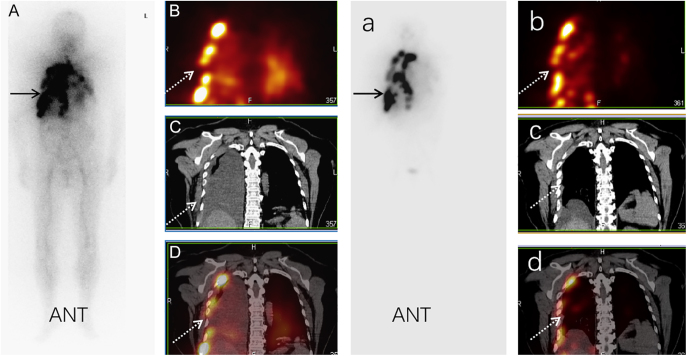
Representative case with a PR. The patient was a 51-year-old woman (case 3) who underwent near-total thyroidectomy with radical right neck dissection for PTC followed by ^131^I therapy at a dose of 7.4 GBq (200 mCi) for pulmonary metastases. A ^131^I-WBS performed 5 days after oral administration of ^131^I revealed diffuse uptake in the chest (A, solid black arrow). A ^131^I-SPECT/CT was then performed to localize the lesions in the chest and indicated uptake of ^131^I in multiple pleural and lung nodules, combined with severe MPE (B, C, D). The patient was retreated with 7.4 GBq of ^131^I for multiple metastases 4 months after the first ^131^I treatment. Post-treatment ^131^I-WBS and ^131^I-SPECT/CT fusion images showed markedly less ^131^I uptake in multiple pleural nodules, indicating a reduction in the lung nodules and MPE (B, C, D). At the same time, the stimulated Tg level decreased from 11,553.00 ng/mL to 1142.00 ng/mL. The patient achieved a PR after four cycles of ^131^I therapy (total dose, 29.6 GBq).
